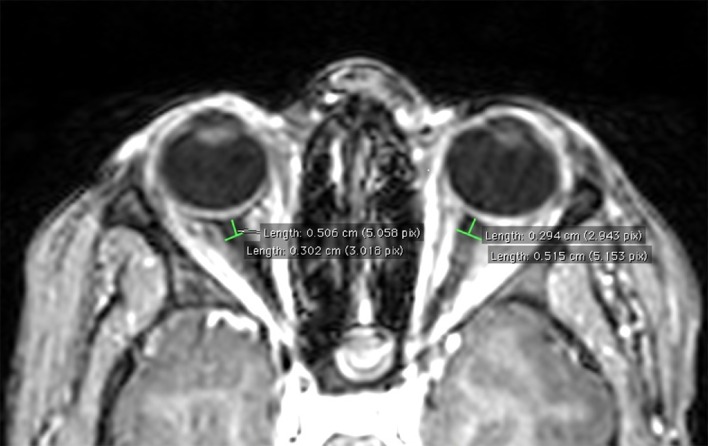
Example of ONSD measurement on T1-weighted magnetic resonance image in a representative subject.
